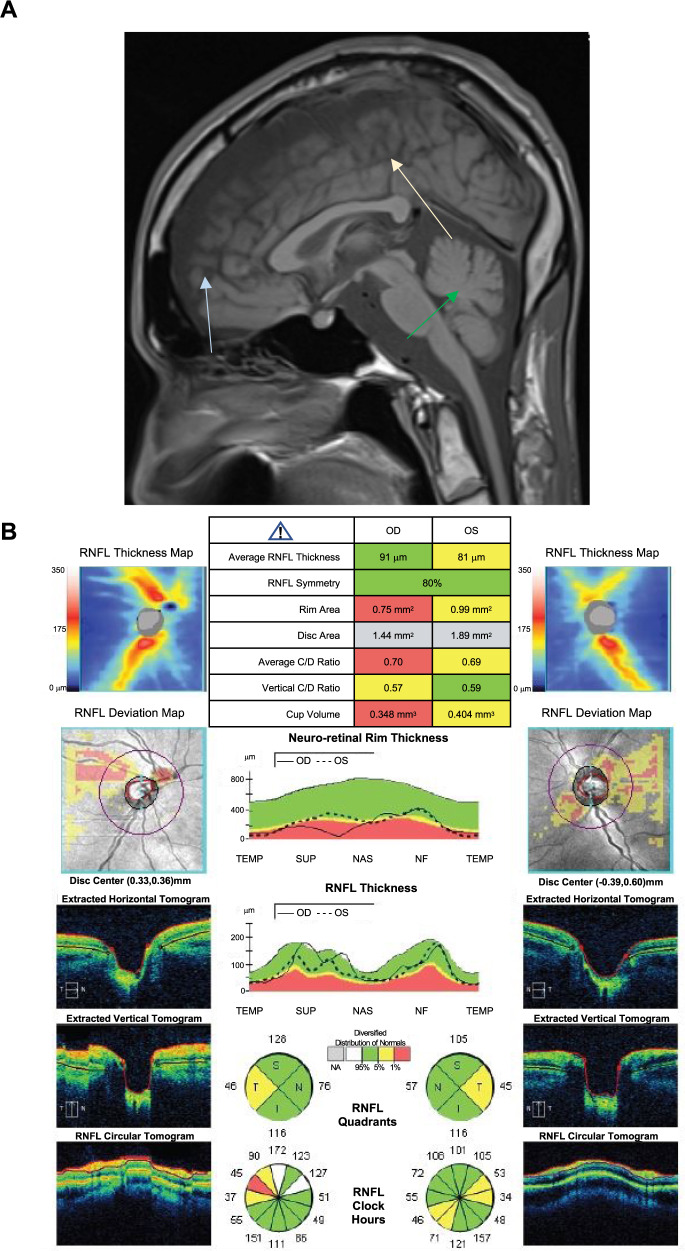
Clinical features of a novel spinocerebellar ataxia variant. A T1-weighted sagittal magnetic resonance imaging (MRI) scan showing mild frontal polymicrogyria (beige arrow), frontal cortical volume loss (blue arrow), and cerebellar atrophy (green arrow). B Optical coherence tomography (OCT) of the optic nerves of proband. On the left-hand side the analysis of the right eye (oculus dexter, OD) is shown, and on the right-hand side, the analysis of the left eye (oculus sinister, OS) is shown. Thinning of the retinal nerve fiber layer (RNFL) is noted in temporal quadrants in both nerves, corresponding to clinically observed optic disc pallor.
